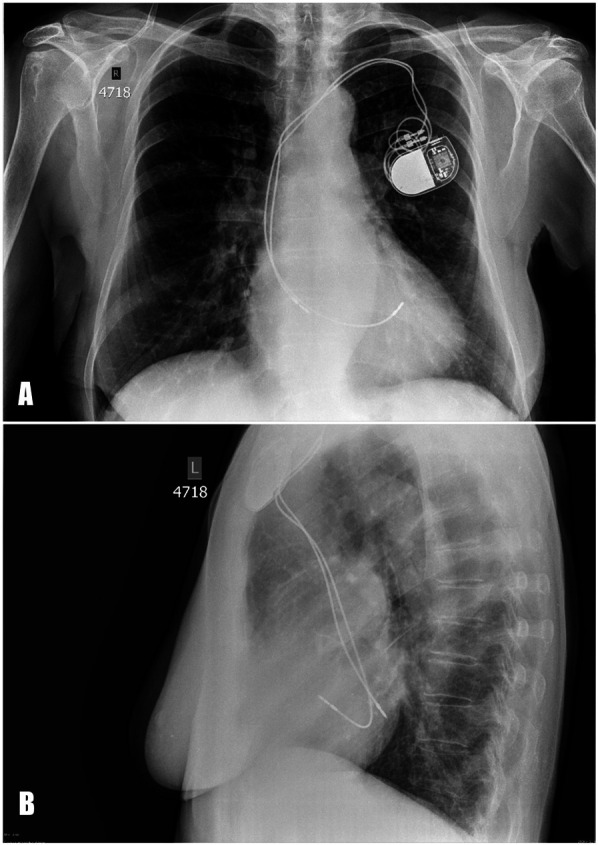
Posteroanterior (А) and lateral (B) chest x-ray. The pacing leads are abnormally positioned, suggesting placement in the left heart instead of the right.
